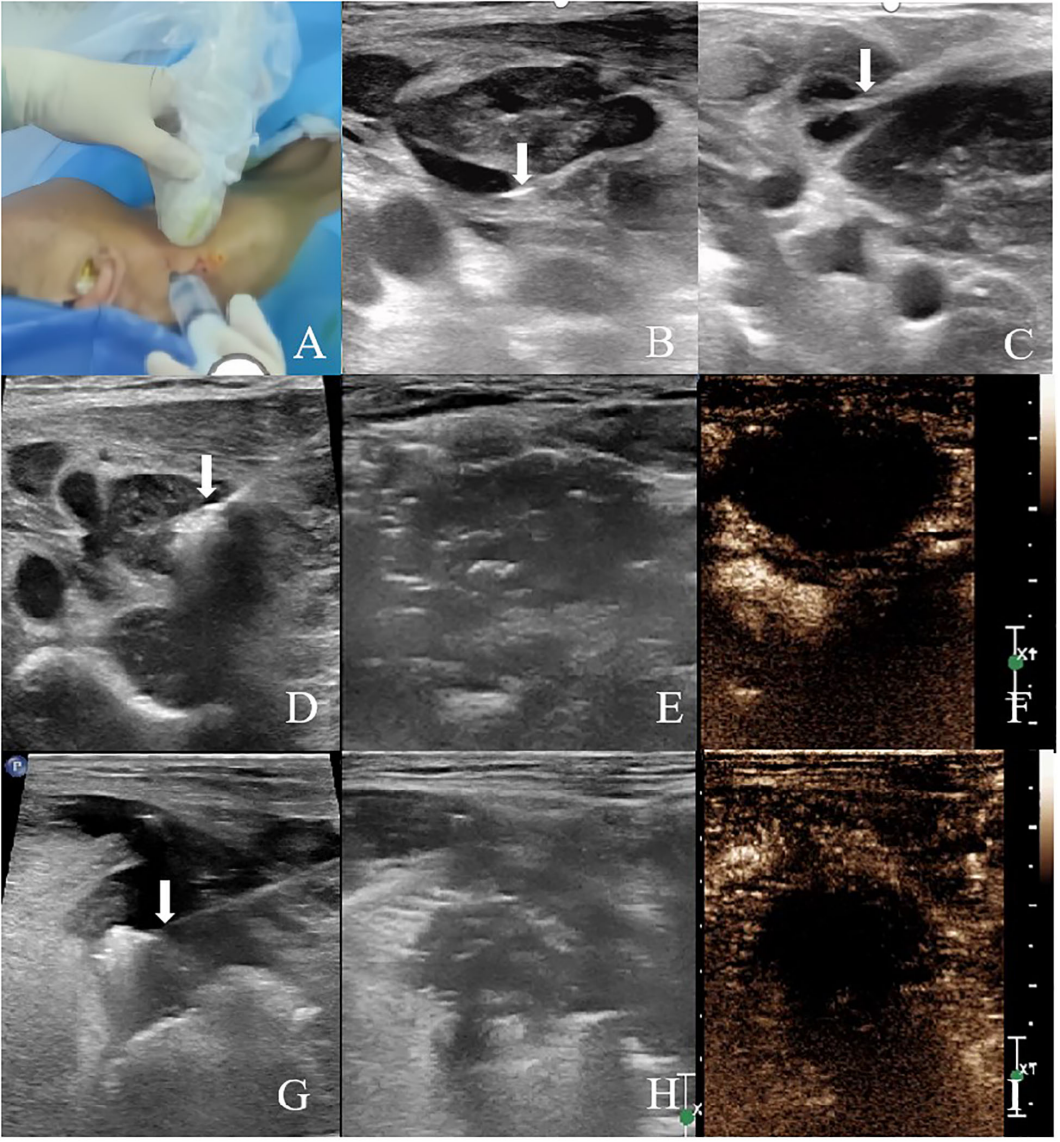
Ultrasound-guided MWA and immediate postoperative CEUS (A–C) Hydrodissection with normal saline was performed prior to MWA to isolate the target lesion. Arrows indicate the tip of the needle. (D) Ultrasound image of MWA of the right cervical lymph node (level II). (E) Two-dimensional ultrasound image of the right cervical lymph node (level II) after ablation. (F) CEUS was performed immediately after ablation, and there was no enhancement in the arterial phase and venous phase of the ablated area of the right cervical lymph node (level II). (G) Ultrasound image of MWA of the right tongue base tumor. (H) Two-dimensional ultrasound image of the right tongue base tumor after ablation. (I) CEUS was performed immediately after ablation, and there was no enhancement in the arterial phase and venous phase of the ablation area of the right tongue base.
